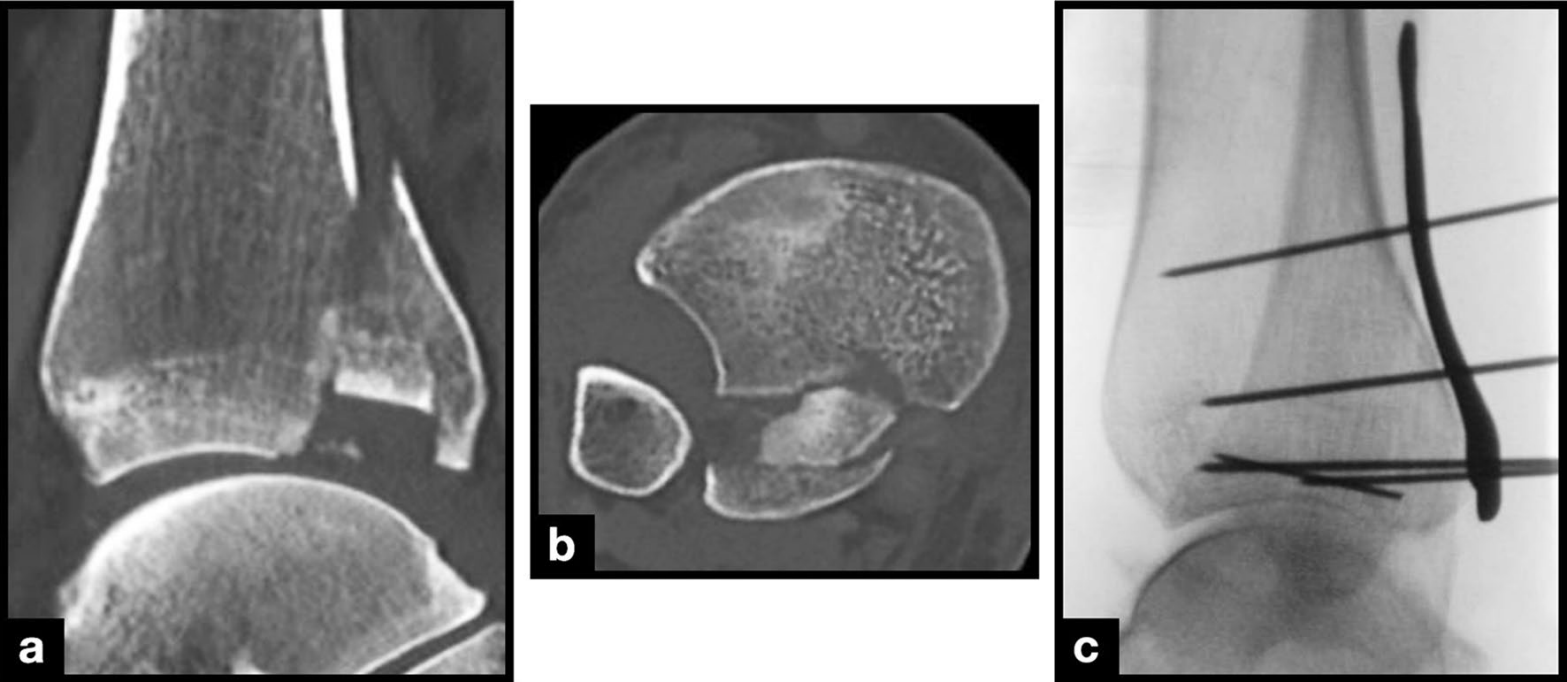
A large intercalary fragment in the a sagittal and b axial plans. c A lateral intraoperative X-ray with fixation of the ICF with a (lost) Kirschner wire (K-wire) and temporary plate fixation with K-wires
